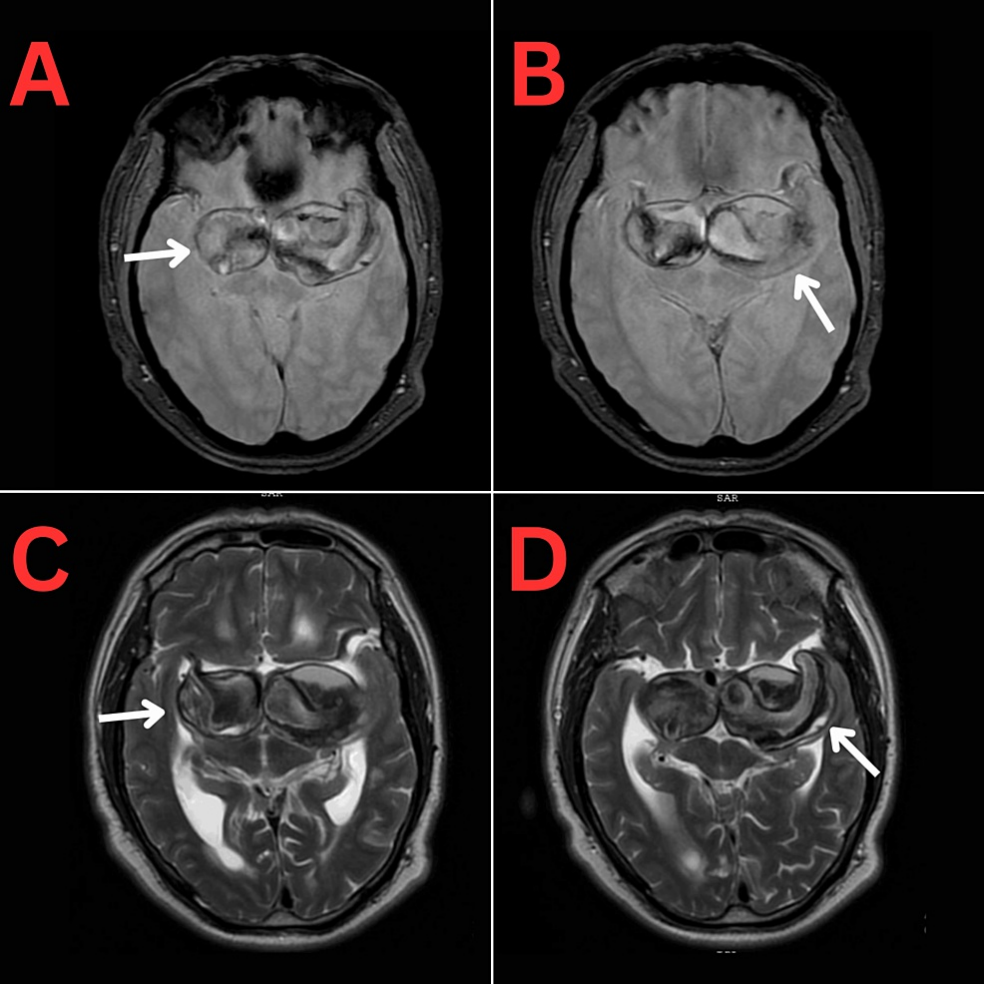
Axial T2-weighted gradient echo (GRE) MRI (A and B) and T2-weighted MRI (C and D) showing the bilateral giant supraclinoid carotid aneurysms (white arrows).
